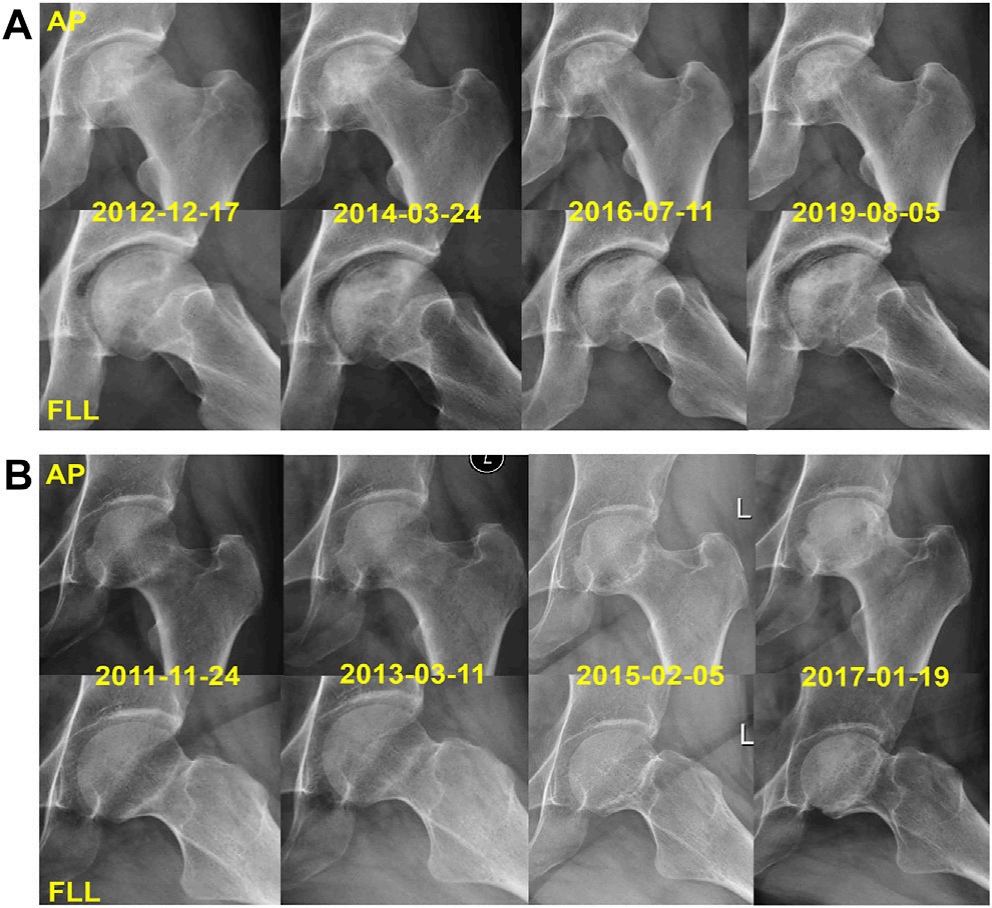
Two representative cases with JIC type C1 lesions. (A) X-rays of the left hip joint of a 42-year-old male with AONFH had a pretreatment LPA of 65° and APA of 67°; there was no collapse of the femoral head in the follow-up of 7.04 years. (B) X-rays of the left hip joint of a 38-year-old female with SONFH had a pretreatment LPA of 57.17° and APA of 59.31°; she has a femoral head collapse in 5.08 years of follow-up.
